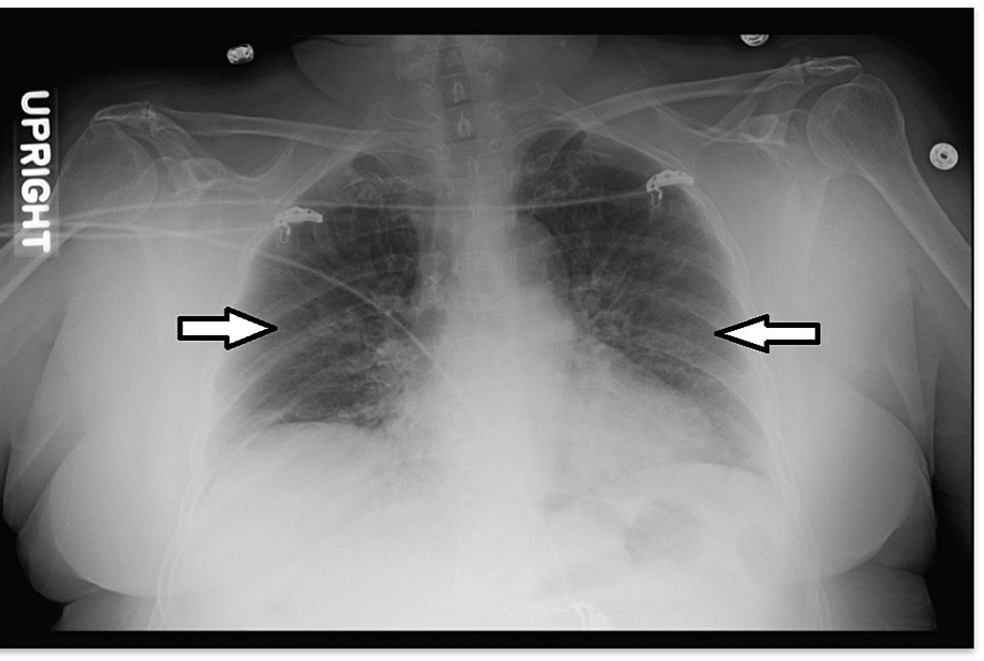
Chest X-ray on admission showing bilateral lung opacities and interstitial markings.
